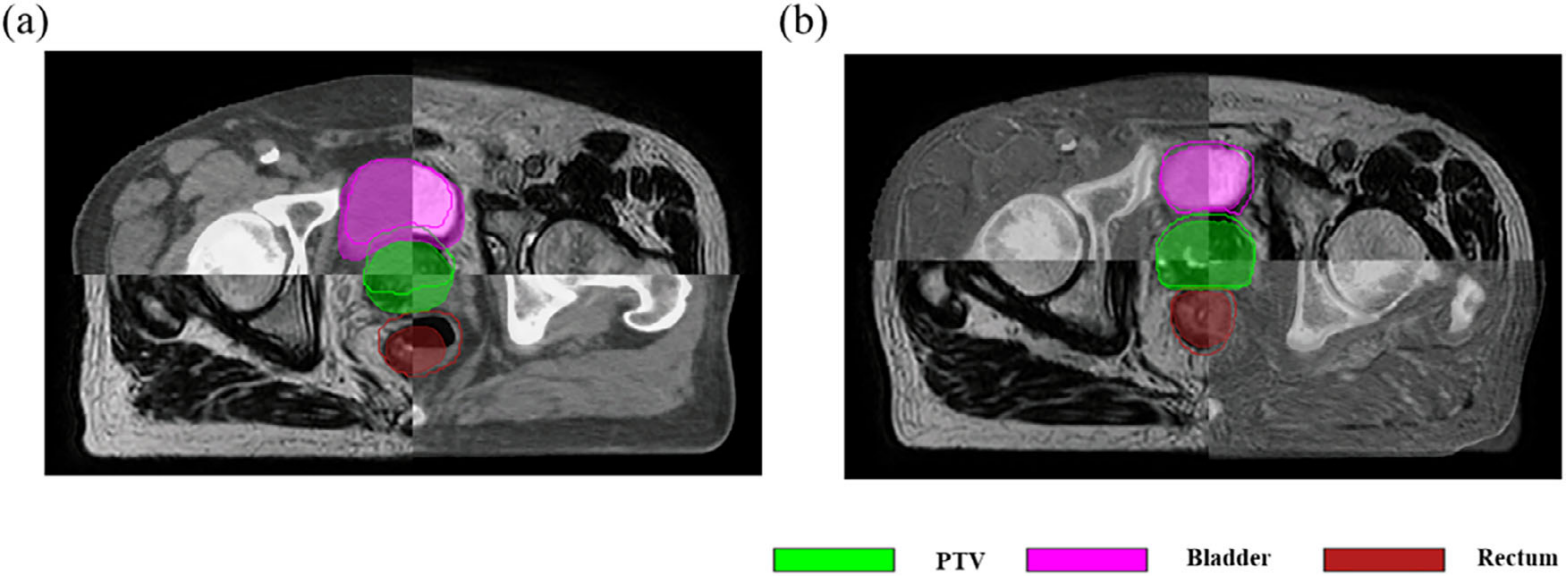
Image registrations for fractions predicted to use the ATS adaptive strategy: (a) Successful case (high deformation); (b) Failure due to registration errors from low soft‐tissue contrast. Contours derived from the reference CT and daily MR are shown as poly lines and color wash, respectively.
